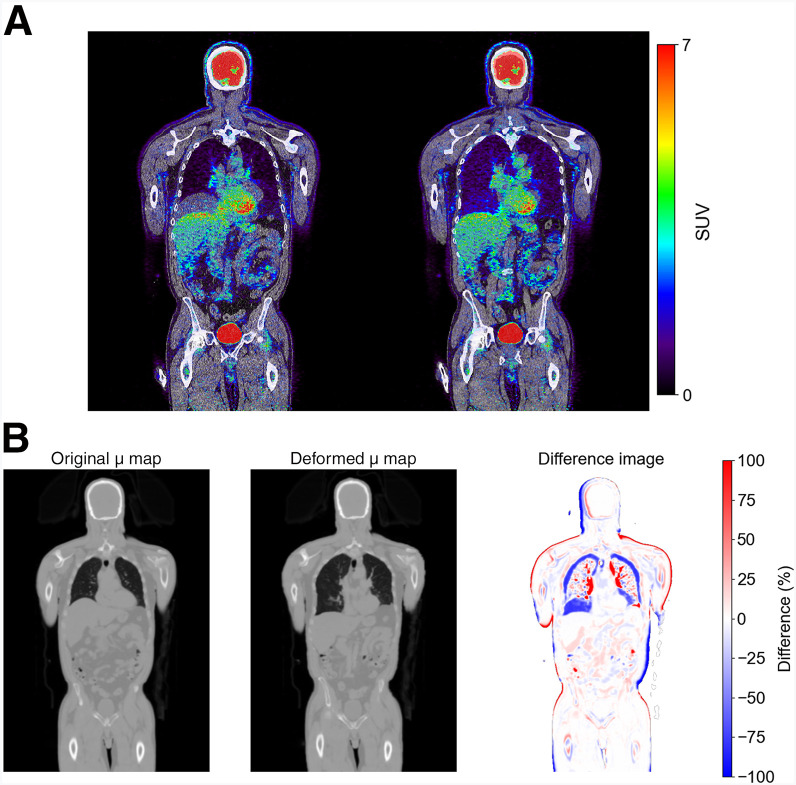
(A) Fused ^18^F-FDG breath-hold PET/CT images reconstructed using original AC-CT (left) and coregistered CT (right). (B) Comparison of μ map with deformed μ map, alongside difference image.
